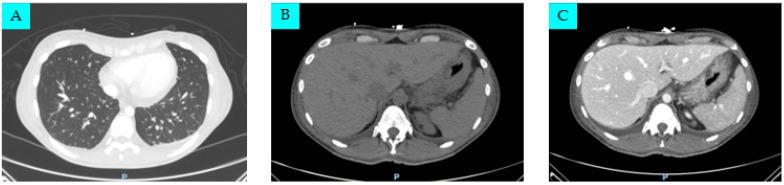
Patient radiological aspect in total body computed tomography (CT) scan: (A) chest CT revealing a 22 mm pericardial effusion and bilateral pleural effusions; (B) abdomen CT revealing severe hepatomegaly and moderate splenomegaly (before contrast); (C) another image of the same abdominal aspect (portal venous phase).
